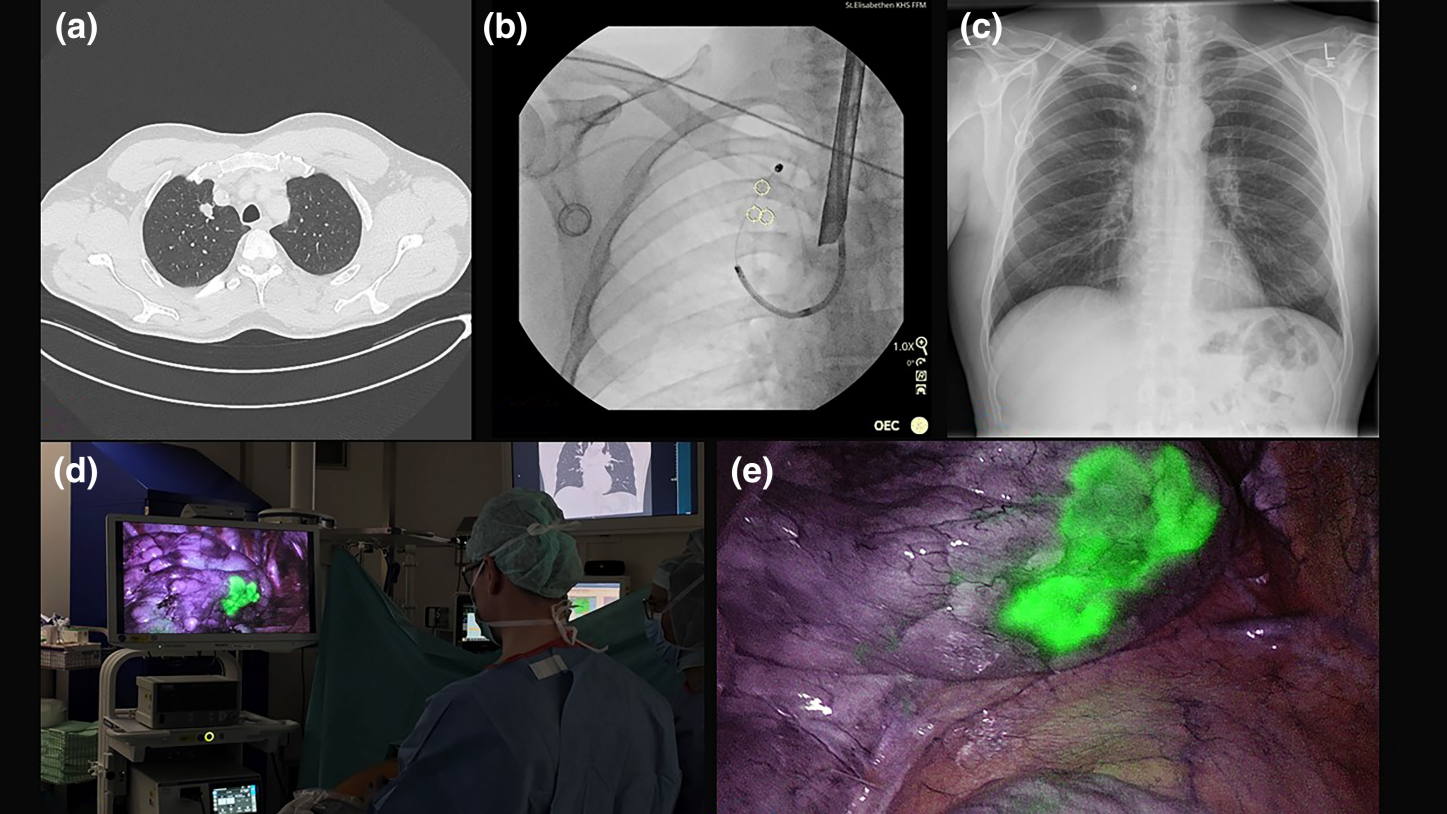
(a) CT scan showing lesion in right upper lobe (S1). (b) Fluoroscopy guided coil deployment in right upper lobe. (c) Postinterventional chest x‐ray showing no pneumothorax and presence of coil in right upper lobe. (d and e) NIR thoracoscopic imaging used to localise the tumour.
